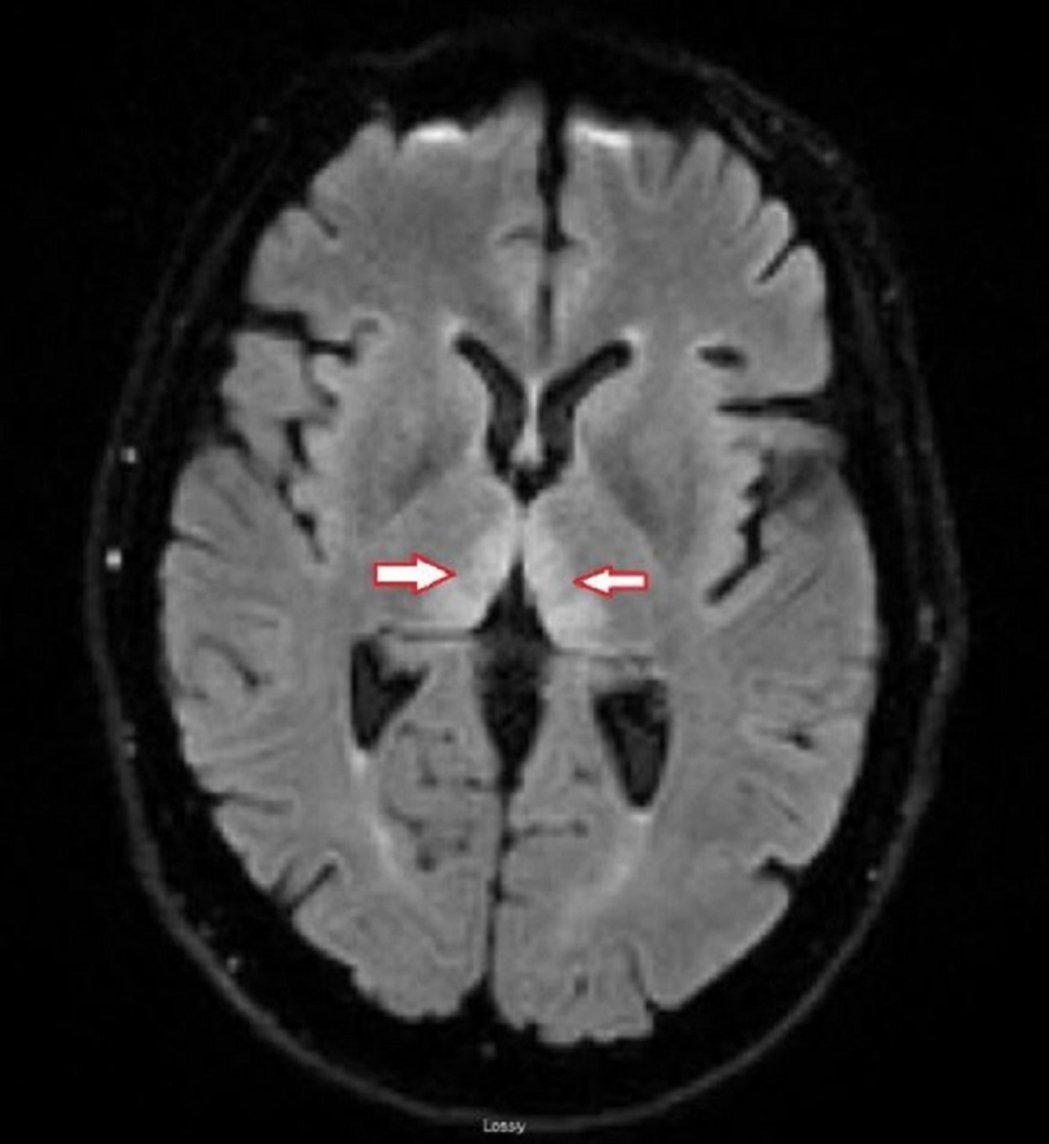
T2/FLAIR sequence of the axial view of the MRI brain shows the bilateral thalamic hyperintensities (red and white arrows) Abbreviations: FLAIR = fluid attenuation inversion recovery; MRI = magnetic resonance imaging
